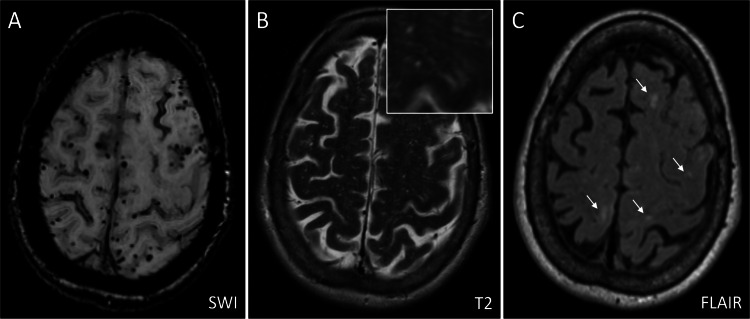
Traditional MRI markers of CAA include lobar cerebral microbleeds and cortical superficial siderosis (A). Recently, white matter features in the form of severe degree of MRI-visible perivascular spaces (B) and white matter hyperintensities in a subcortical multi-spot pattern (C, arrows) were added to the version 2.0 of the Boston criteria for the clinical diagnosis of CAA during life. SWI: susceptibility-weighted imaging, FLAIR: fluid-attenuated inversion recovery
